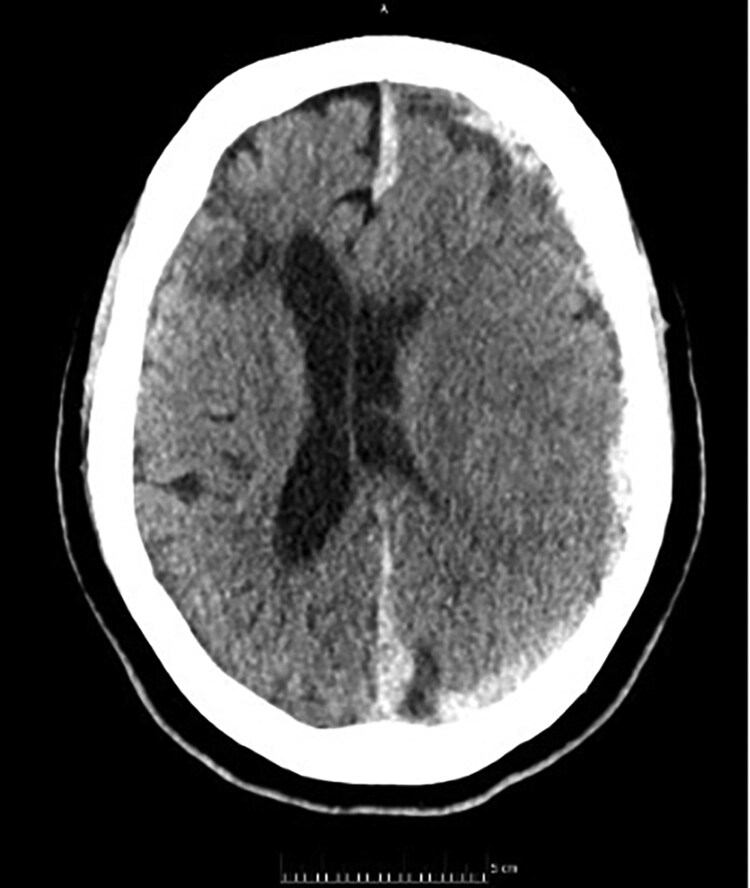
Computed tomographic imaging of the brain on presentation with dysphasia and confusion 7 months post-operatively, showing a left subdural haematoma with mass effect (midline deviation).
